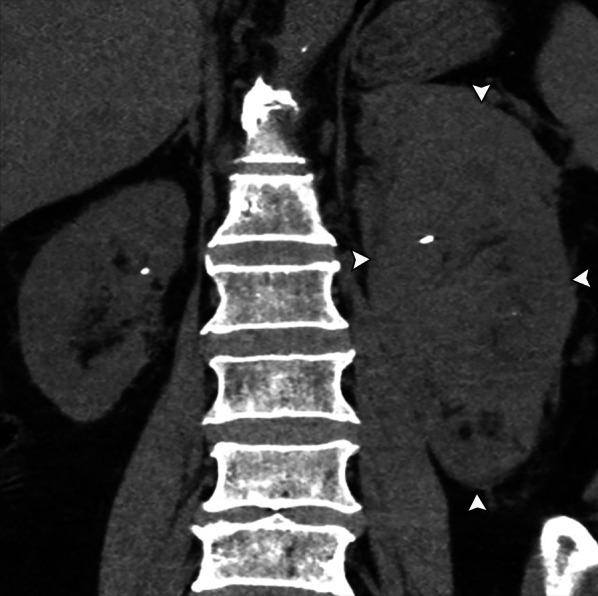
Nephromegaly of renal lymphoma.* Coronal noncontrast CT demonstrates infiltrative renal lymphoma with a diffusely enlarged left kidney replaced by tumor (arrowheads). Note the encasement and deformity of the pelvocalyceal system by tumor. This was pathologically confirmed as marginal zone lymphoma
